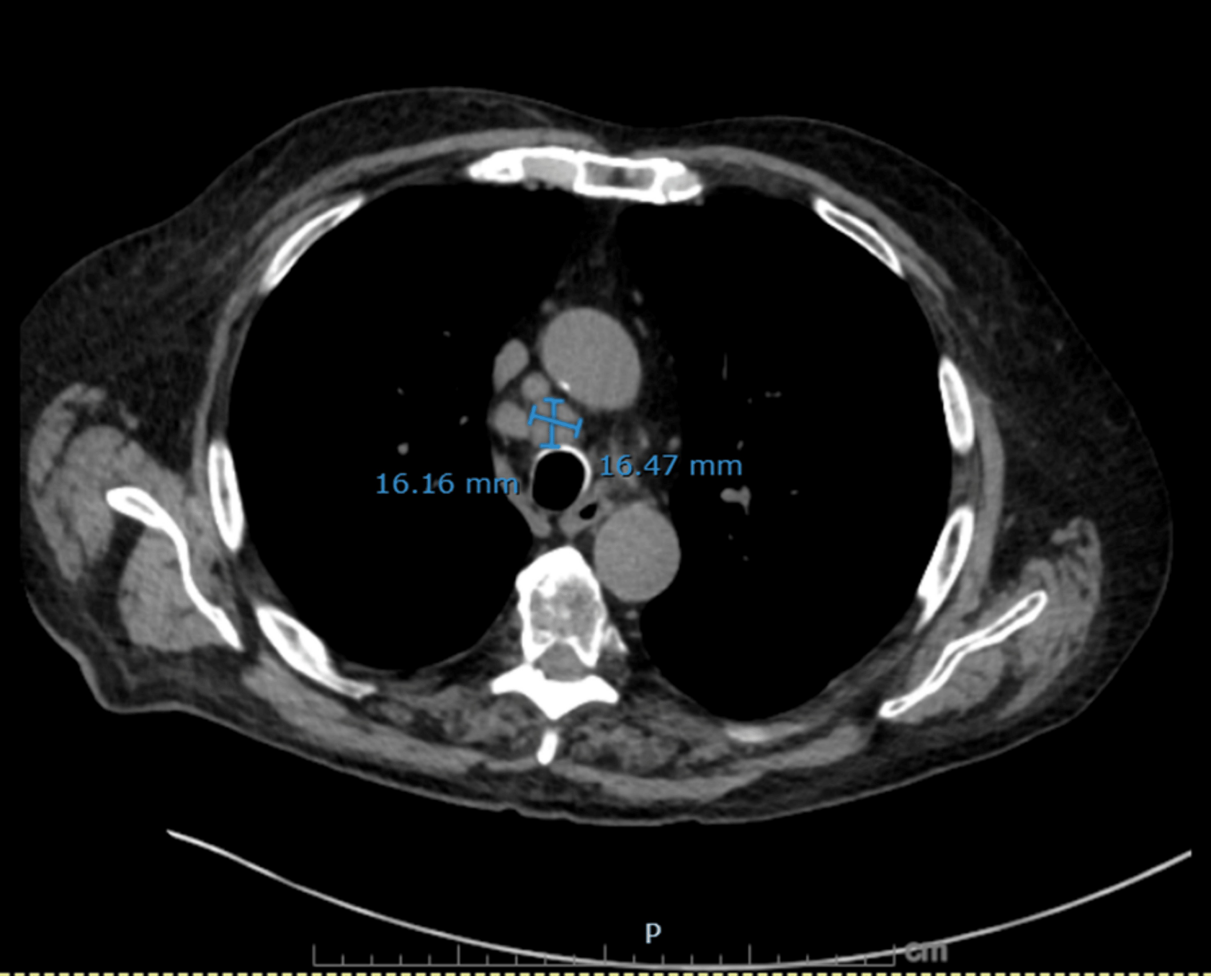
Non-contrast axial CT chest showing a dominant right paratracheal lymph node A right paratracheal lymph node measuring 16.2 × 16.5 mm is demonstrated, further supporting malignant mediastinal involvement in small cell lung cancer.
